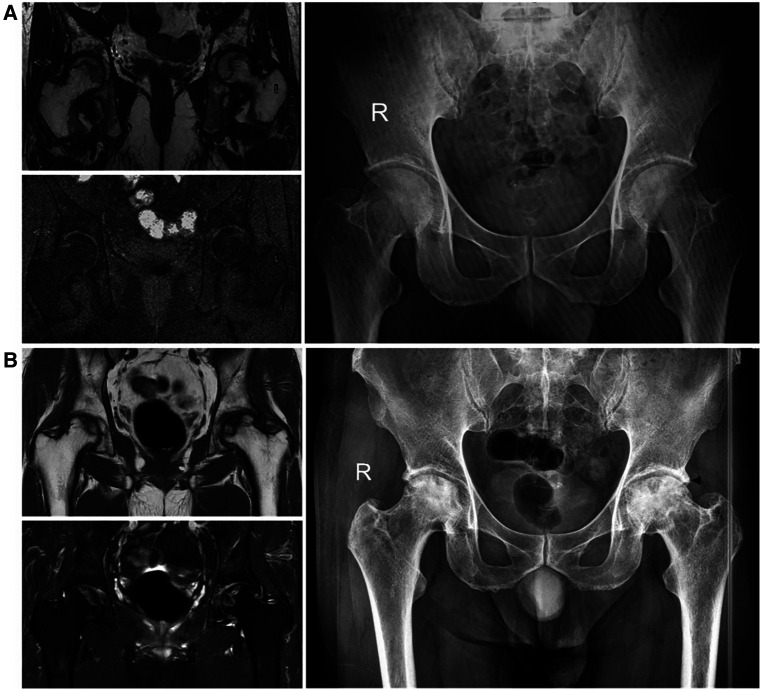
The presentive MRI (T1WIs and T2WIs) and x-ray films at diagnosis and endpoint. (A) Imaging at diagnosis: a limited subchondral linear-shaped low signal intensity in T1WIs and a “double-line sign” in T2-weighted images in MRI and an AP view of bilateral hips, no signs of collapse in x-ray films (ARCO Stage 1). (B) Imaging at the endpoint: subtle signs of osteosclerosis (right, ARCO Stage 2), fracture in the subchondral zone and femoral head depression ≤2 mm (left, ARCO Stage 3A), mottled appearance of femoral head and osteosclerosis in bilateral hips in x-ray films. The black arrowhead shows the collapse position.
